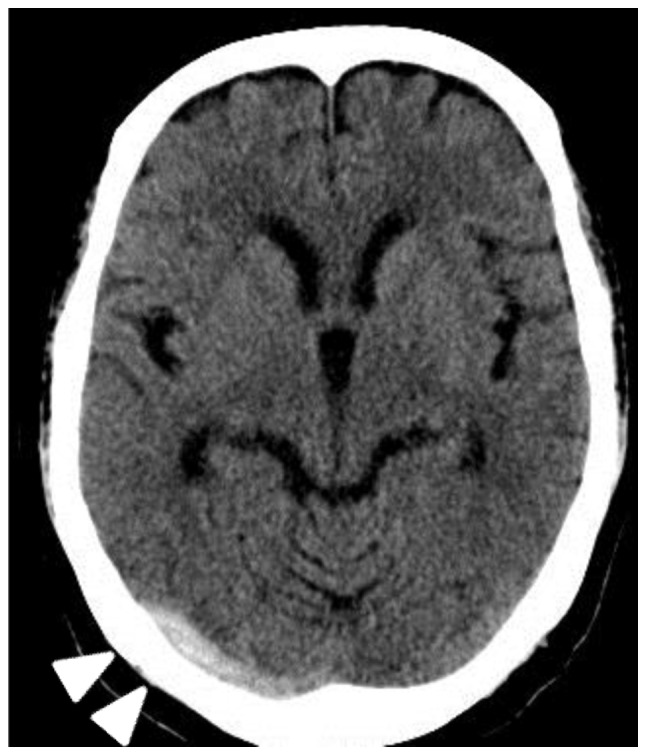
Computed tomography on the 33rd day of hospitalization. A hyperdense area at the right transverse venous sinus is shown (arrowheads).
